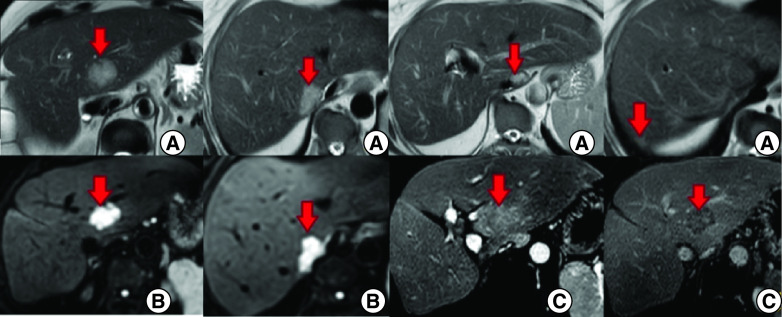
Hepatic MRI – axial sections. (A & B) Hepatic lesions (red arrows) in hypersignal T2 and diffusion respectively. (C) Post-contrast images show enhancement in the arterial phase with a wash out in the portal and late phases.
